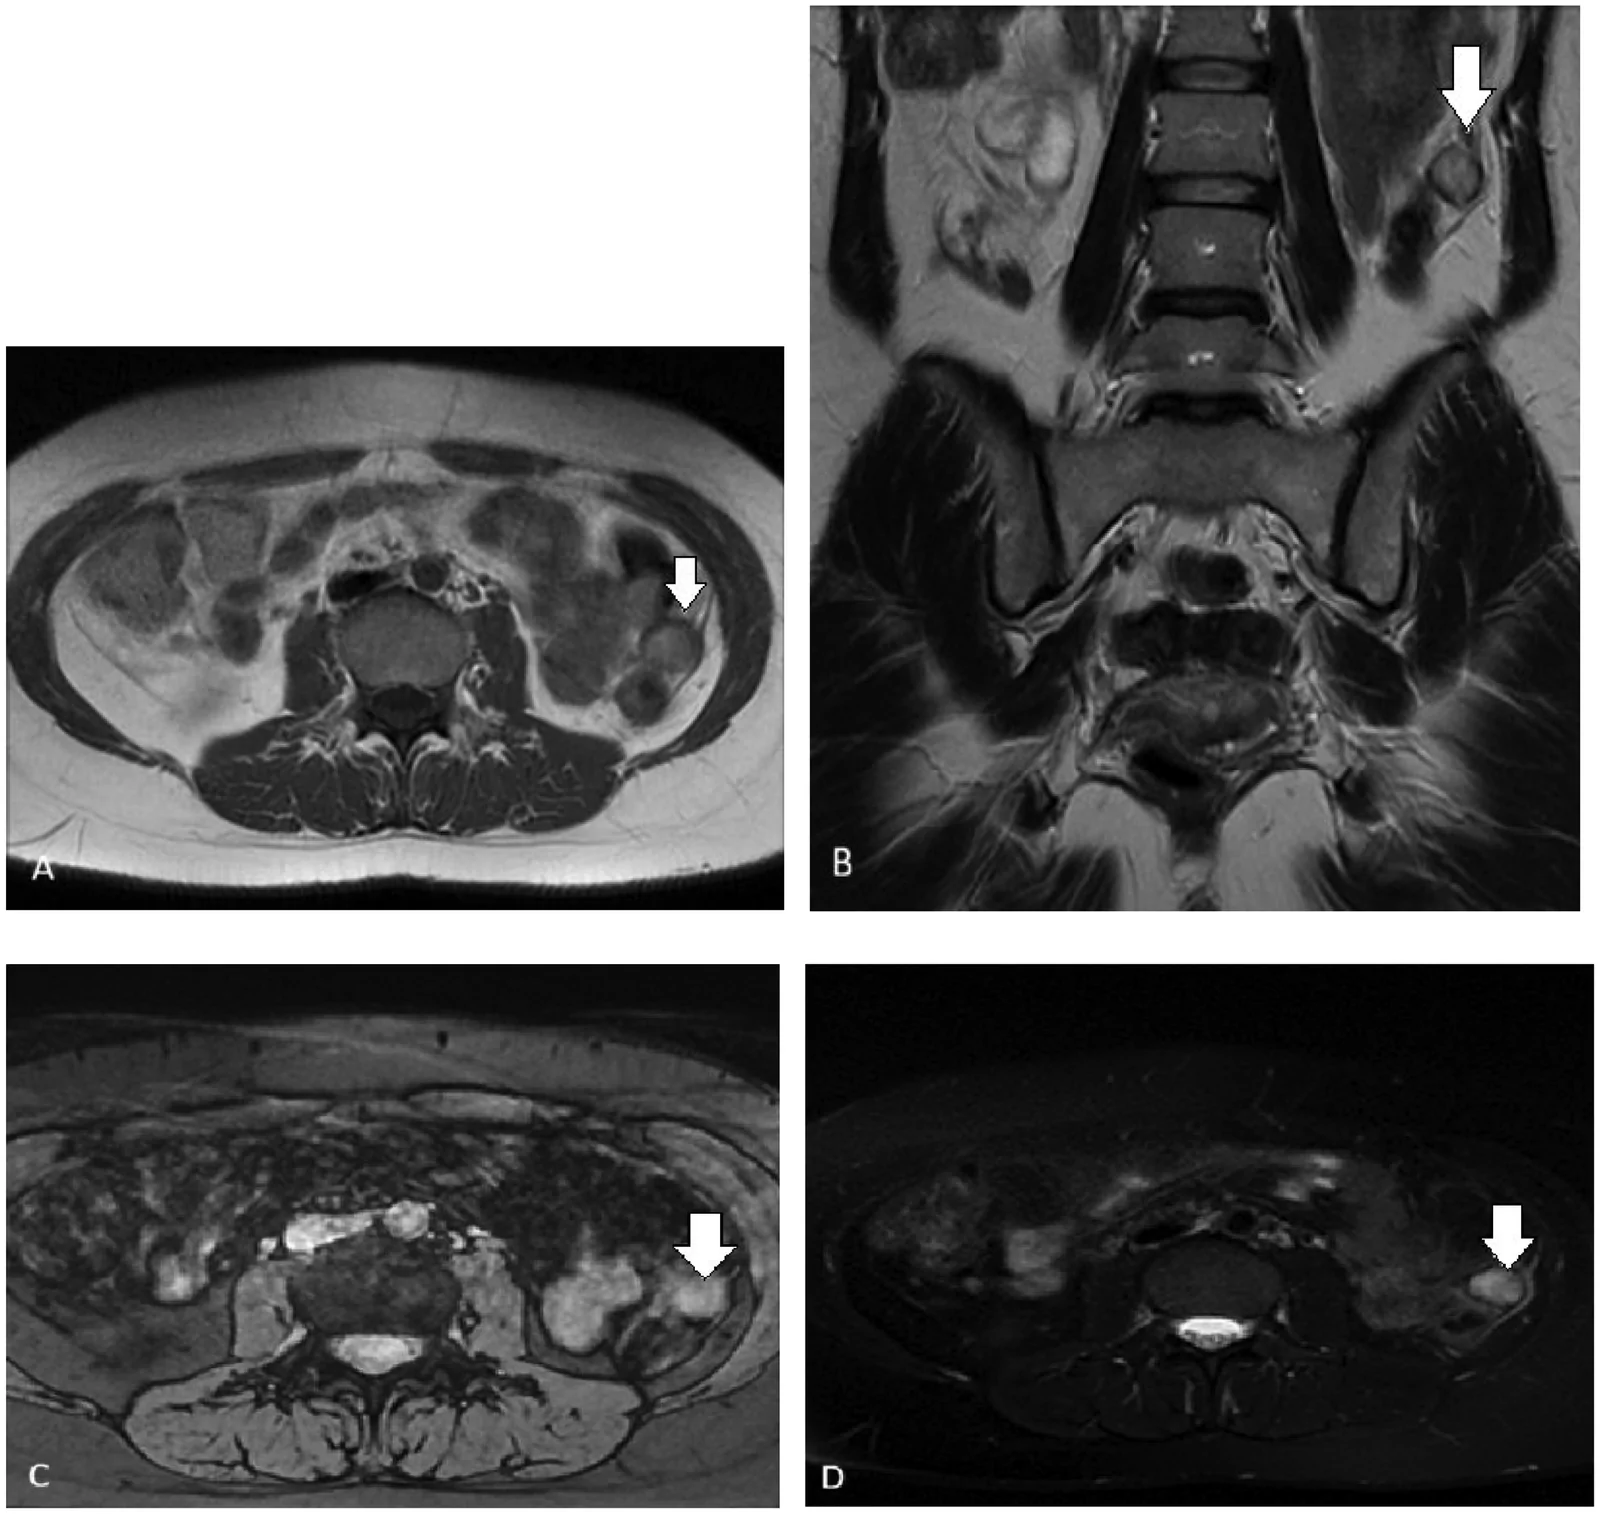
MRI of the abdomen and pelvis. (A) Axial T1WI and (B) Coronal T2WI showing a cystic nodule adjacent to the descending colon, exhibiting intermediate to hyperintense signal on both sequences. (C) Axial T2* GRE WI showing no blooming artifacts. (D) Axial T2FS WI revealing a persistent hyperintense signal on the fat suppression sequence.
